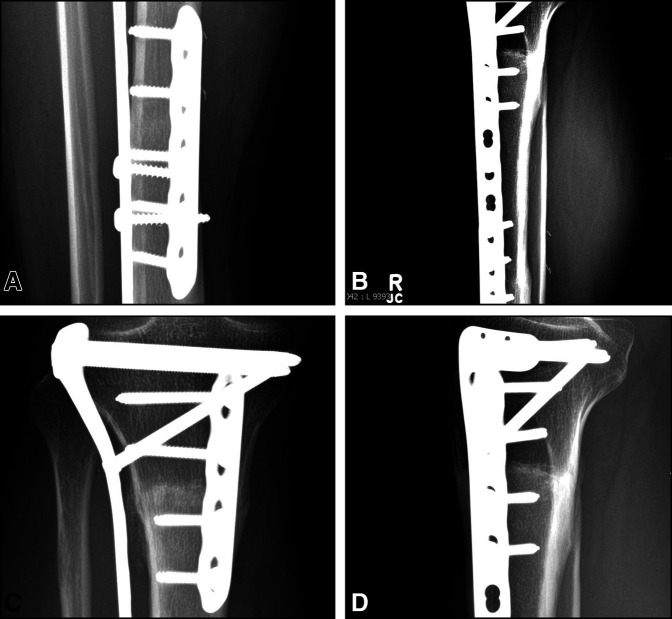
Radiograph showing AP and lateral views. A, Case 1: AP radiograph of distal tibia demonstrating host-allograft junction union at the 33-month follow-up. B, Case 1: lateral radiograph of distal tibia diaphyseal host-allograft junction demonstrating union at the 33-month follow-up. C, Case 1: AP radiograph of proximal tibia demonstrating host-allograft junction union at the 33-month follow-up. D, Case 1: lateral radiograph of proximal tibia demonstrating host-allograft junction union at the 33-month follow-up.
